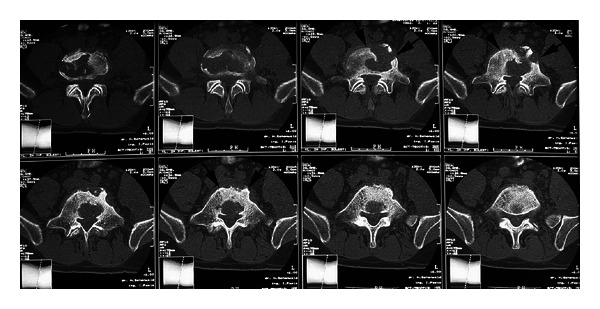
CT scans demonstrated permeative bone destruction of the lower end plate of the fourth lumbar vertebra and the upper end plate of the fifth lumbar vertebra, consistent with spondylodiscitis in a patient with “unmasking” tuberculosis- (TB-) associated immune reconstruction inflammatory syndrome (TB-IRIS) (arrows) (Case no. 2).
